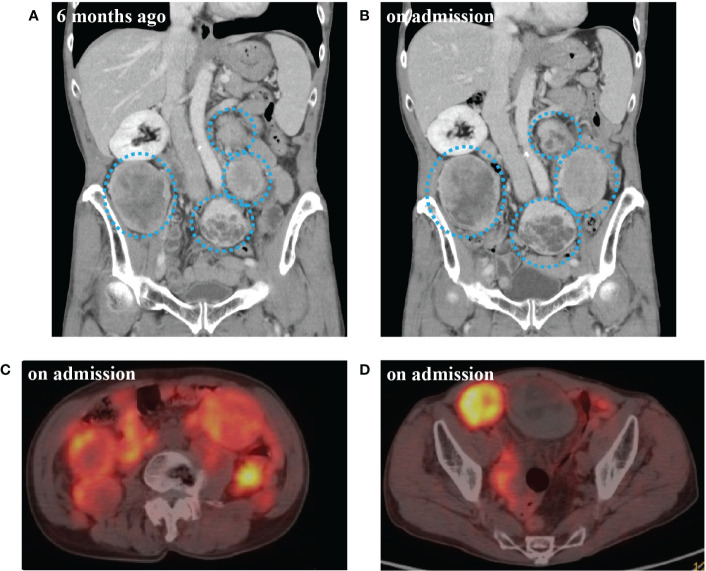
(A) Computed tomography (CT) scan of abdomen 6 months before admission. (B) CT scan at the time of admission. (C)
